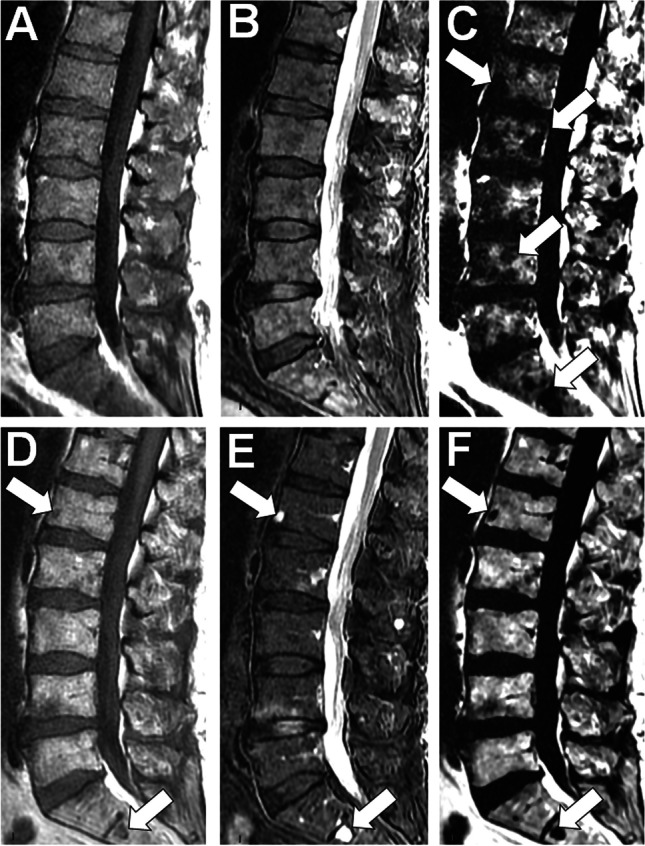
73 year-old man with newly diagnosed MM (IgG G kappa, stage III, ISS 1): MRI findings at baseline and post ASCT. A, B, C Baseline sagittal T1- (A) and T2 Dixon Water Only (B) and Fat Only (C) MR images of the lumbar spine show diffuse heterogeneous and relatively low signal intensity of the bone marrow on T1, with heterogeneous nodular high signal intensity on the Water Only image. Foci of marrow replacement are evident on the Fat only image (arrows in C), underestimated on the T1 image probably to the relatively high signal of myeloma foci on this sequence. D, E, F Post-treatment corresponding MR images obtained 12-m later after high dose cytotoxic treatment and ASCT show almost complete return to normal signal intensity of the bone marrow on the different sequences. Note however the persistence of rare foci of low signal on the T1 and Fat Only images and very high signal on the Water Only image (arrows in D, E, F)
